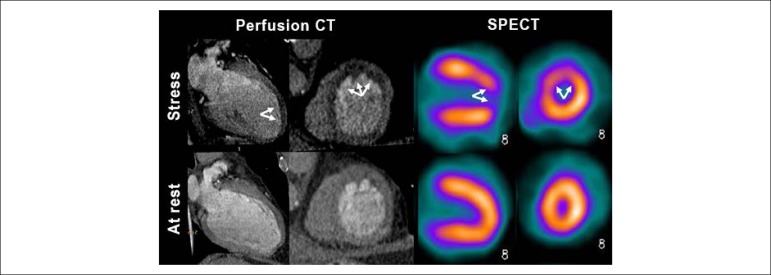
Comparison between myocardial perfusion images with stress perfusion defects on computed tomography (CT) and on single-photon emission computed tomography (SPECT). Concordant example of a same patient with significant obstructive anterior descending (LAD) coronary artery disease.
